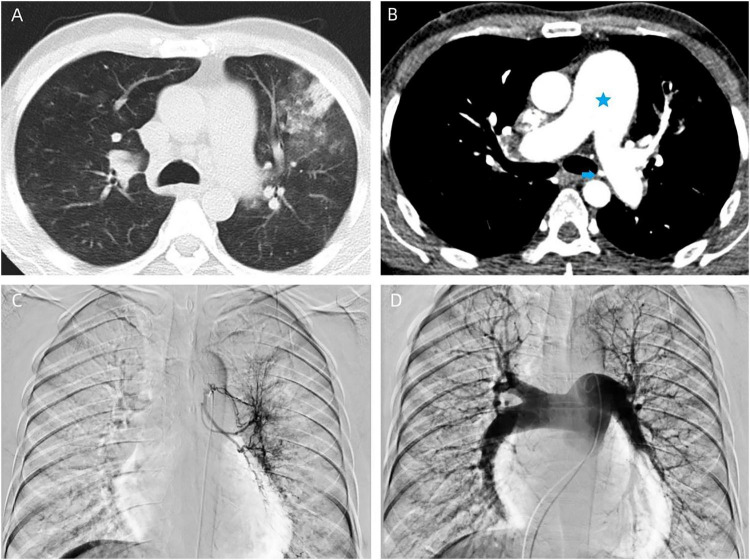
Imagings of the case. (A) CT scan shows effusions in the left lung. (B) CT angiography reveals dilated pulmonary arteries (star) and tortuous bronchial arteries (arrow). (C) Bronchial artery angiography demonstrates dilated and tortuous bronchial arteries. (D) Pulmonary artery angiography reveals dilation of the main and bilateral pulmonary arteries, with sparse visualization of distal pulmonary arteries.
